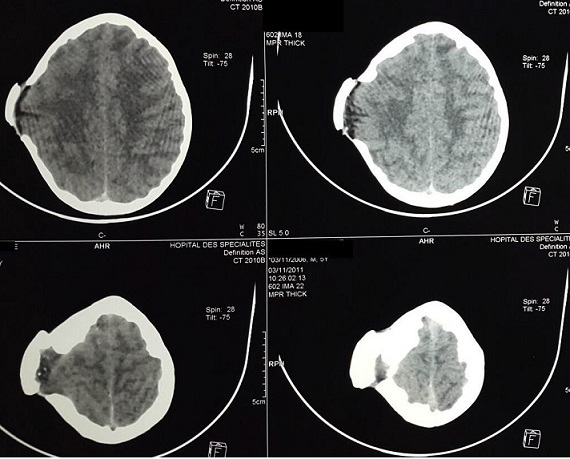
Radiographie du crâne simple de profil objective des empreintes digitiformes diffuses avec aspect d'ex-barrure pariétale et synostose des sutures coronales
